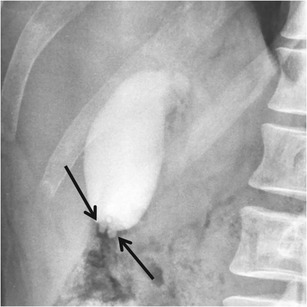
Gallbladder adenomyomatosis: typical oral cholecystography findings. In this case of fundal type GA, RAS (*arrows*) are filled by contrast material as a consequence of their communication with the gallbladder lumen. Courtesy of Marco Ferigato, radiographer at Bolzano Central Hospital
